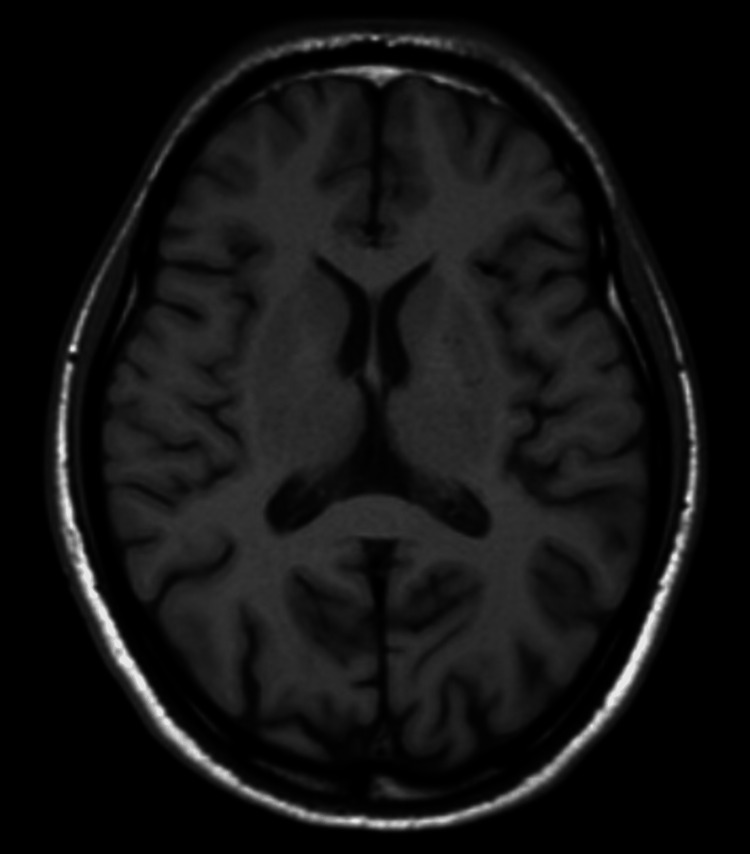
Normal brain MRI (T1-weighted imaging) with no mass effect. Relative volume loss is seen in other sections.
